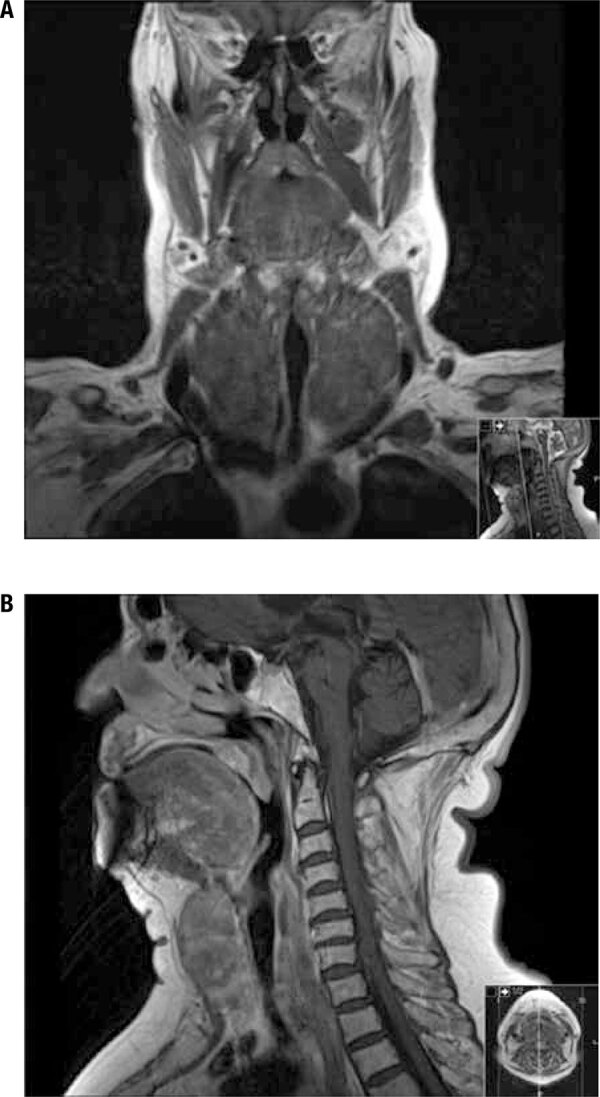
Magnetic resonance imaging of the head and neck showing compression of the trachea by a multinodular goiter. (A) Coronal view. (B) Sagittal view.
